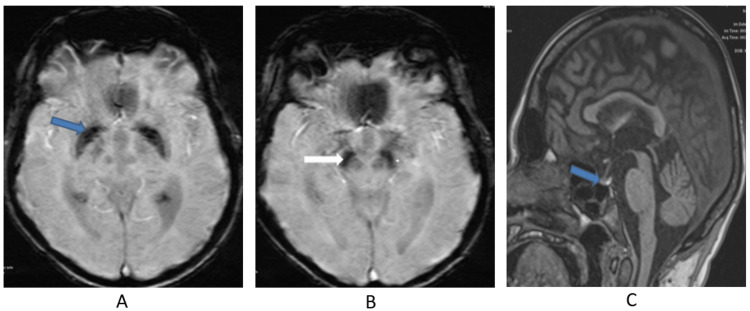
Images A and B are axial susceptibility weighted imaging (SWI) of the brain showing putaminal blooming artifacts reflecting iron accumulation and iron deposition in the substantia nigra (blue and white arrows). Image C is a sagittal T1-weighted MRI showing a partially empty sella and a small pituitary gland (blue arrow).
